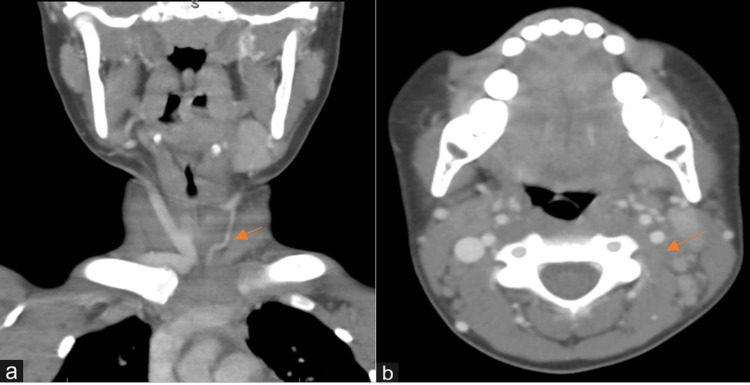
A neck computed tomography slices showing areas of thrombophlebitis (arrows) of left internal jugular vein. (a) Coronal view of the neck CT. (b) Horizontal section of the neck CT.
